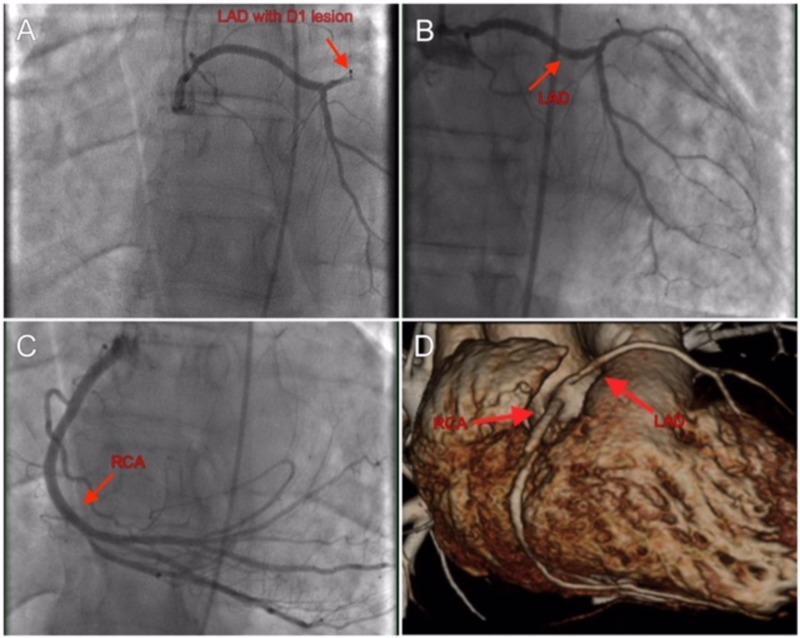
Angiographic findings and cardiac computed tomography (CT) imaging of the anomalous vessels. (A) Left anterior descending artery with 100% D1 lesion. (B) Culprit vessel post intervention. (C) Super-dominant right coronary artery. (D) Cardiac CT showing origin of RCA (left) and LAD (right) from right sinus of Valsalva. RCA: Right coronary artery; LAD: Left anterior descending artery.
